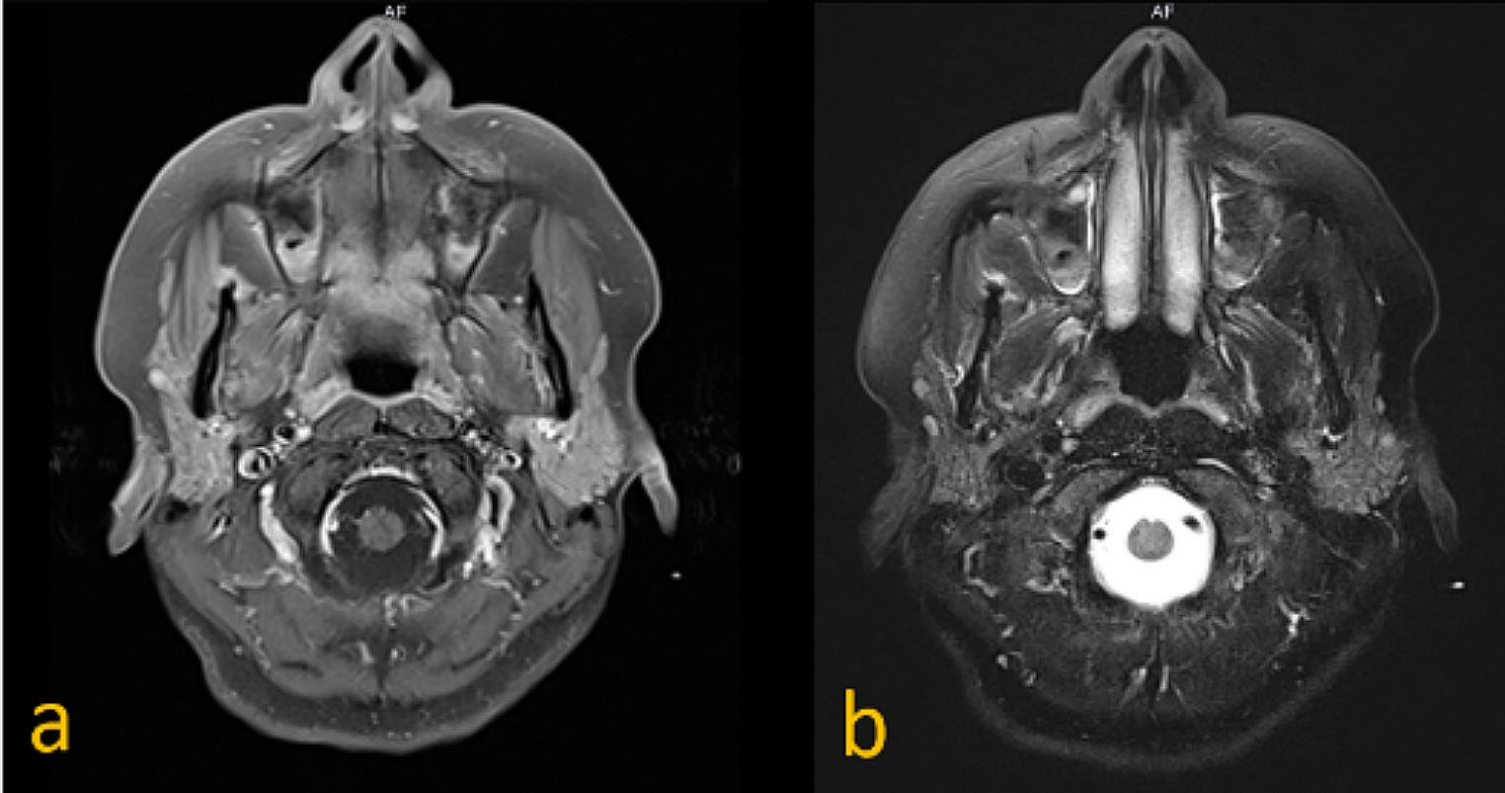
(a) MRI, T1, transversal; (b) MRI, T2, transversal. The right parotid gland shows even less parotid gland tissue then the left parotid gland. No lesions are depictable
